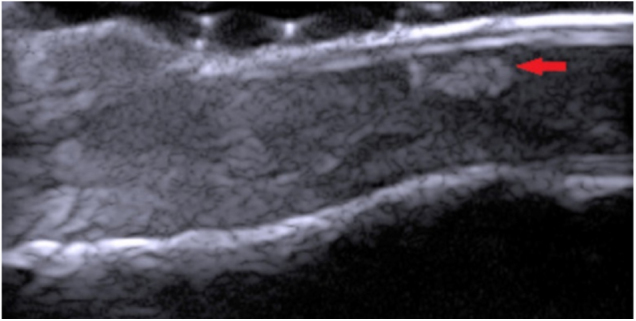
HFUS, 22 MHz, longitudinal view (Case 3). Hyperechogenic, heterogeneous area with irregular shape (arrow), avascular, located in the nail bed with Doppler mapping.
